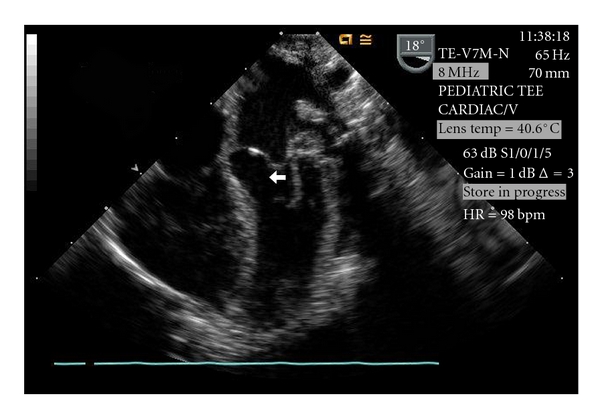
Post-evacuation TEE demonstrating no residual DVSH and a widely patent LVOT. Key: DVSH—dissecting ventricular septal hematoma, LVOT—left ventricular outflow tract, TEE—transesophageal echocardiogram.
